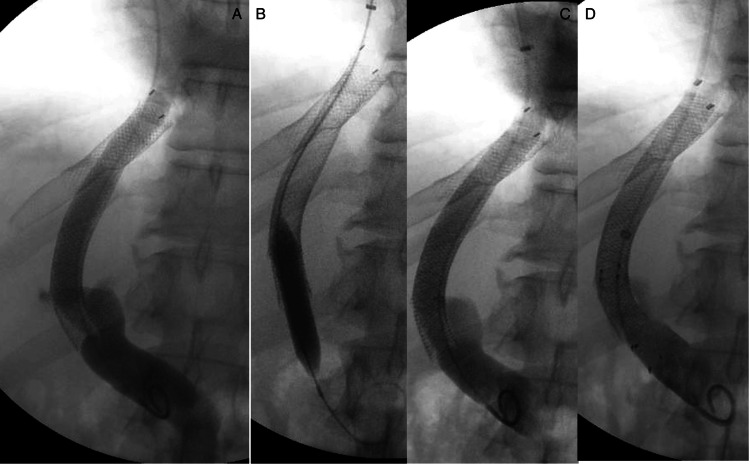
Outpatient TIPS revision: (A) initial cavogram; (B) angioplasty; (C) post-angioplasty; (D) post-stent. A 59-year-old man with alcohol cirrhosis status post TIPS eight years prior, TIPS revision six years prior, and new-onset ascites. TIPS Doppler velocity of 202 cm/second. (A) High-grade stenosis in the inferior stent graft with HVPG 24 mmHg. (B) and (C) Failed 10 mm balloon angioplasty of the stenosis, HVPG 16. (D) 10 mm x 40 mm bare metal stent placement, HVPG 14. Ascites resolved within one month, and the TIPS remained functional in follow-up for five years. TIPS, transjugular intrahepatic portosystemic shunt; HVPG, hepatic venous pressure gradient
